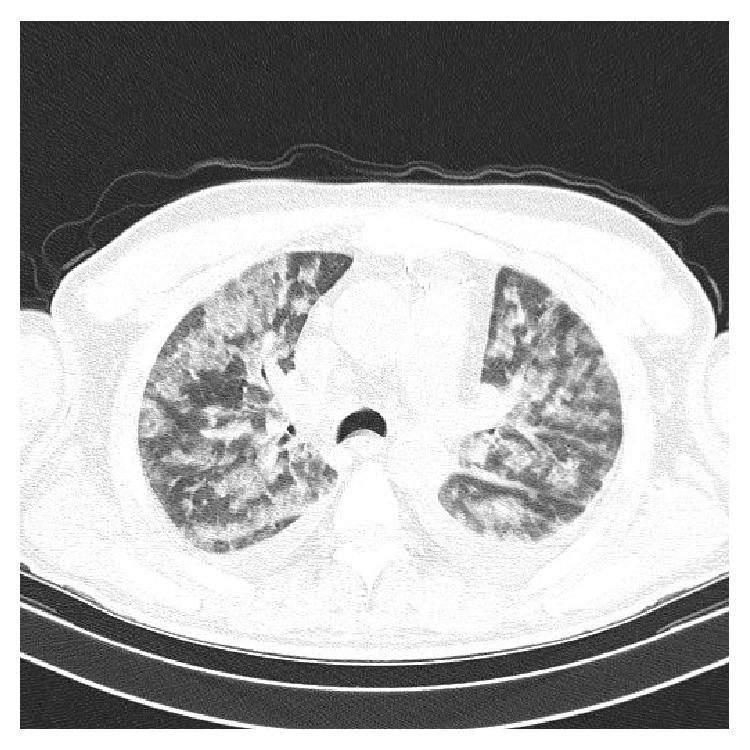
Computed tomography of the chest on admission. High resolution CT of the chest showed bilateral patchy airspace disease.
